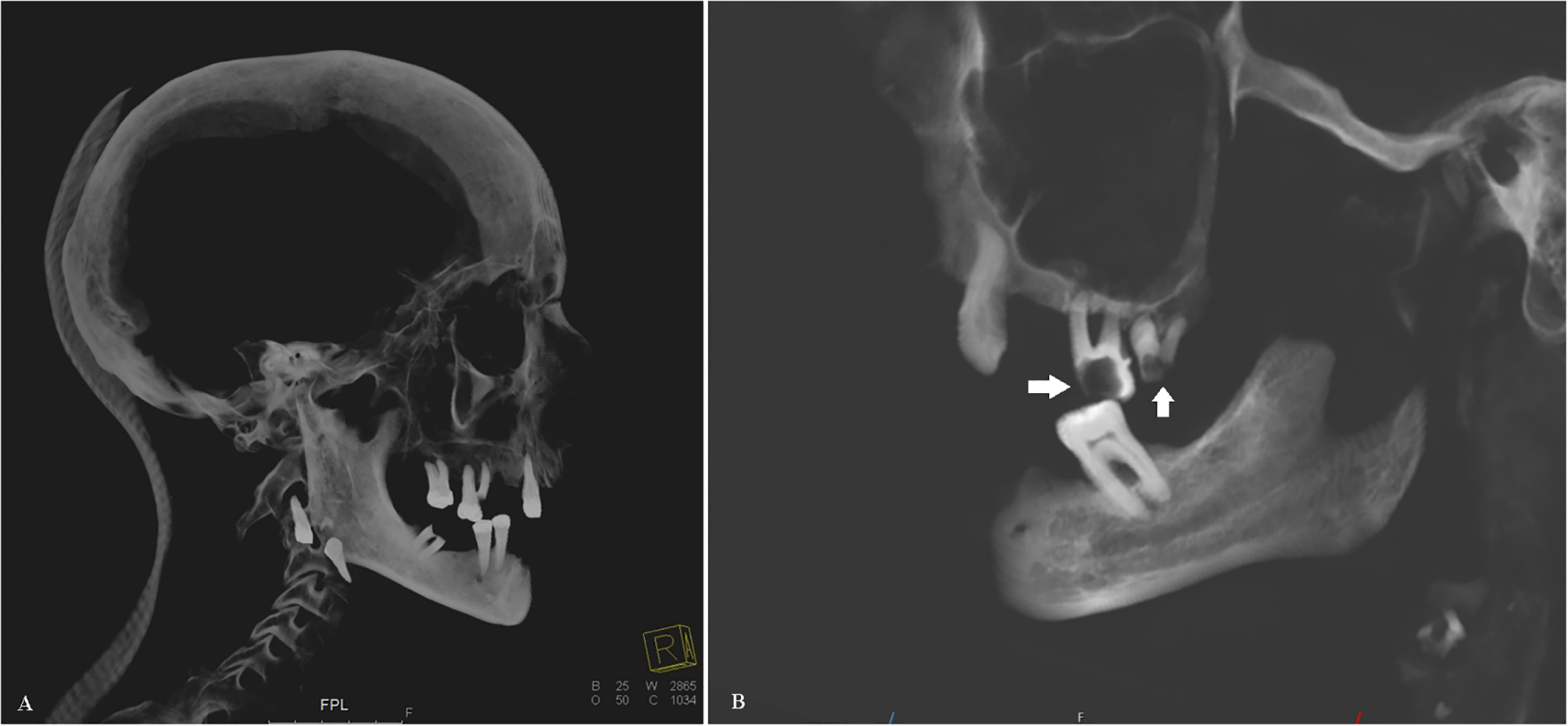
Sagittal multi-planar MIP reconstruction of a CT representing the skull of individual D. (A) The dentition shows several alterations including ante-mortem tooth loss, carious lesions, destructive periodontal processes, and post-mortem displacement of teeth. (B) Detail of the dentition showing ante-mortem tooth loss and severe carious lesions (arrows).
